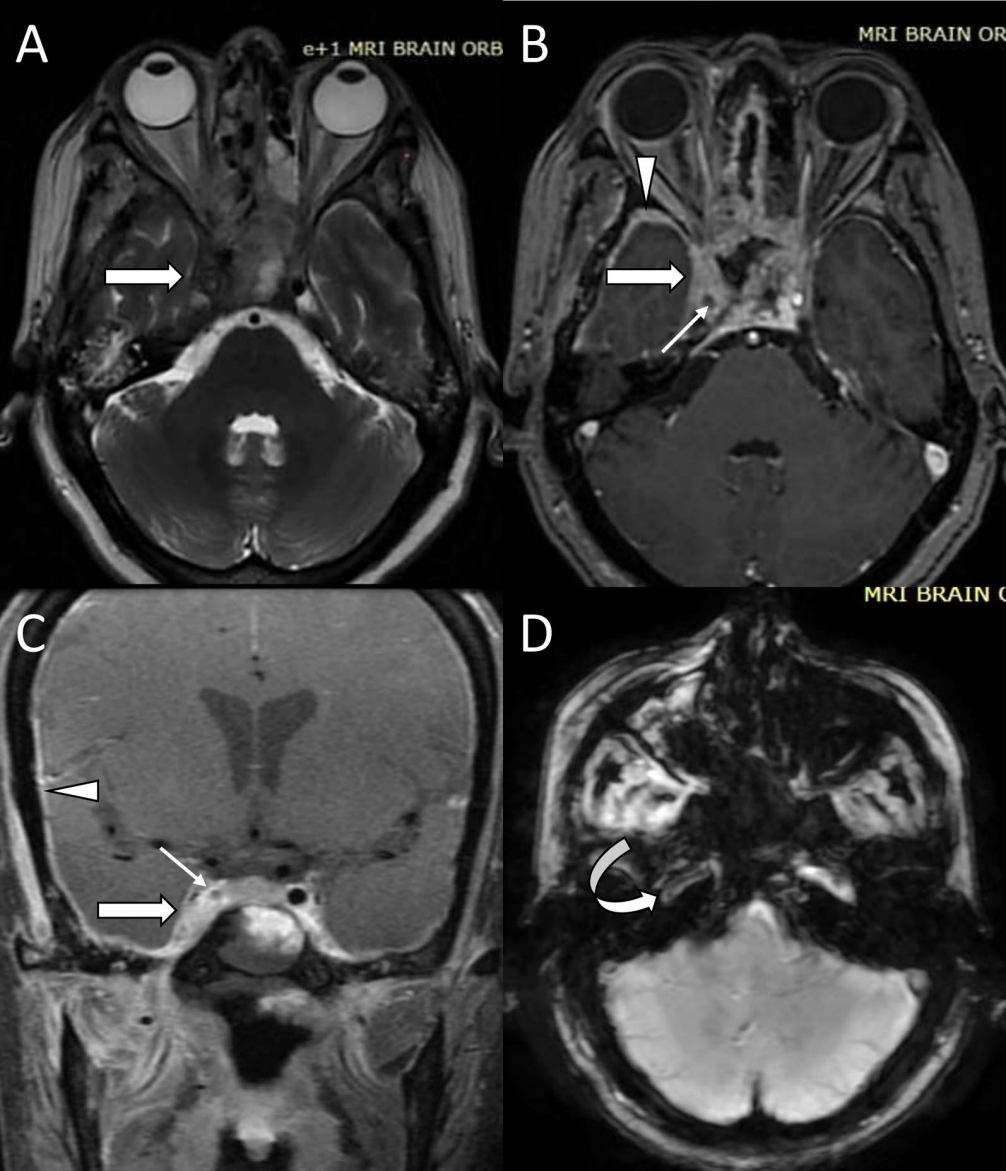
Right cavernous sinus (CS) and internal carotid artery (ICA) thrombosis. Axial T2-weighted (A), contrast-enhanced axial (B), and coronal (C) T1-weighted fat-saturated images show the bulging lateral border of the right cavernous sinus (white large arrows) with loss of normal flow void of the right internal carotid artery (white small arrows). The right CS shows filling defects on contrast-enhanced images indicating its thrombosis. Note the thickened and enhancing dura (white arrowheads) adjacent to the right temporal lobe. Left intracranial ICA shows normal flow void. Susceptibility-weighted image (D) shows blooming in the petrocavernous part of the right ICA suggestive of the presence of thrombus
